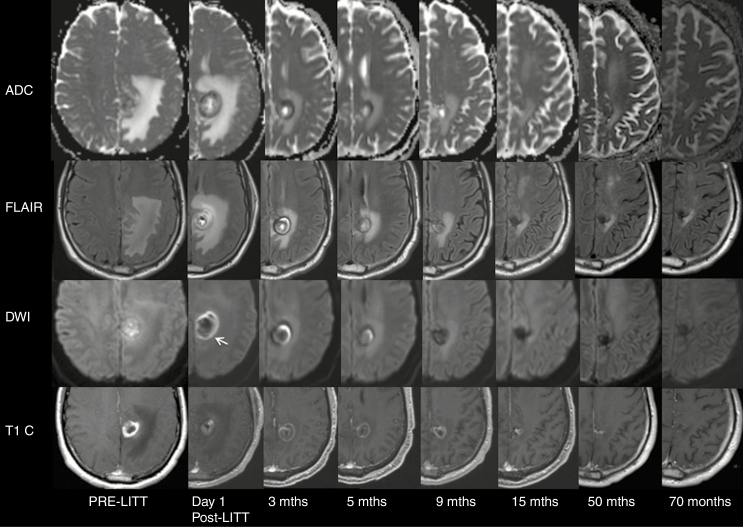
MR imaging (ADC maps, T2 FLAIR, DWI, and T1 postcontrast images) in a representative case without recurrence of a left parietal GBM treated with LITT. At day 1 post-LITT, there is a complete undisrupted periphery of decreased ADC and DWI hyperintensity (white arrow) at the edge of the treatment zone that marks the border of the peripheral rim of enhancement (representative border shown, not all images are included in this figure). The peripheral restricted diffusion shrinks exponentially parallel to the peripheral enhancement and was resolved after 9 months. Contrast enhancement decreased gradually in the first 15 months, stable up to 5 years and resolved after 70 months.
